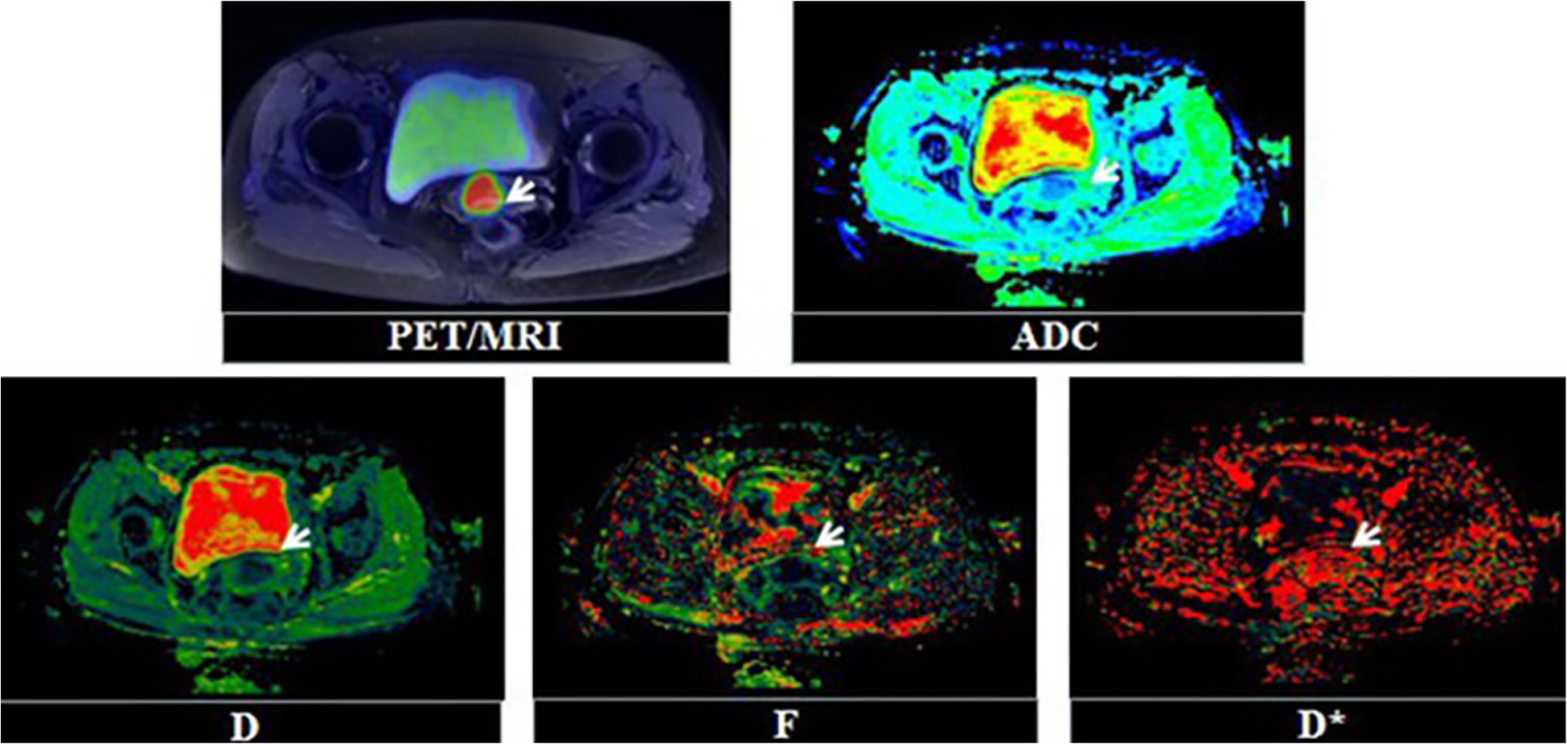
Fifty-nine years-old female patient diagnosed moderately differentiated, squamous cervical carcinoma, with no suspected lymph node metastasis showed on preoperative PET/MR, but it showed metastatic on postoperative pathology. The primary tumor has concentrated FDG uptakes on PET/MR fusion images (SUV_max_ = 11.68 g/cm^3^, SUV_mean_ = 7.92 g/cm^3^, TLG = 79.99 g, MTV = 10.1 cm^3^), and the ADC and IVIM image showed a high signal in the tumor (ADC_mean_ = 1.21 × 10^− 3^ mm^2^/s, ADC_min_ = 0.7 × 10^− 3^ mm^2^/s, D_mean_ = 0.87 × 10^− 3^ mm^2^/s, D_min_ = 0.5 × 10^− 3^ mm^2^/s, D* = 56.93 × 10^− 3^ mm^2^/s, f = 0.17)
